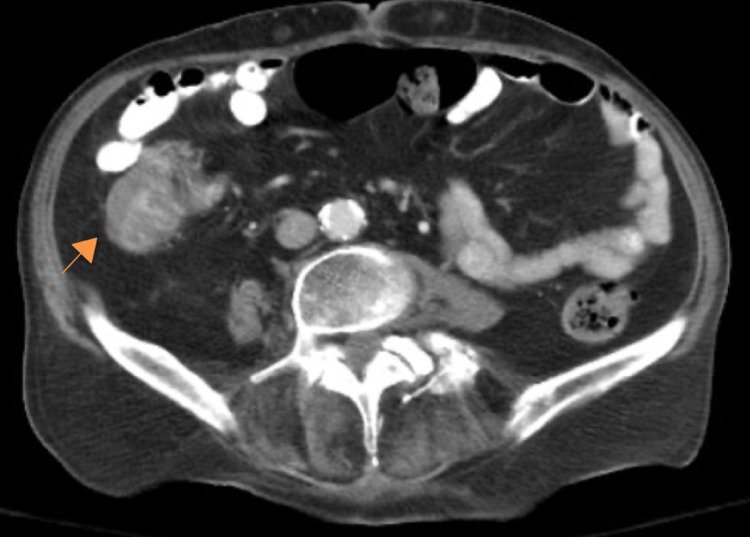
Axial CT image, with both IV and PO contrast, demonstrating thickened inflammatory changes in the ascending colon (orange arrow)
